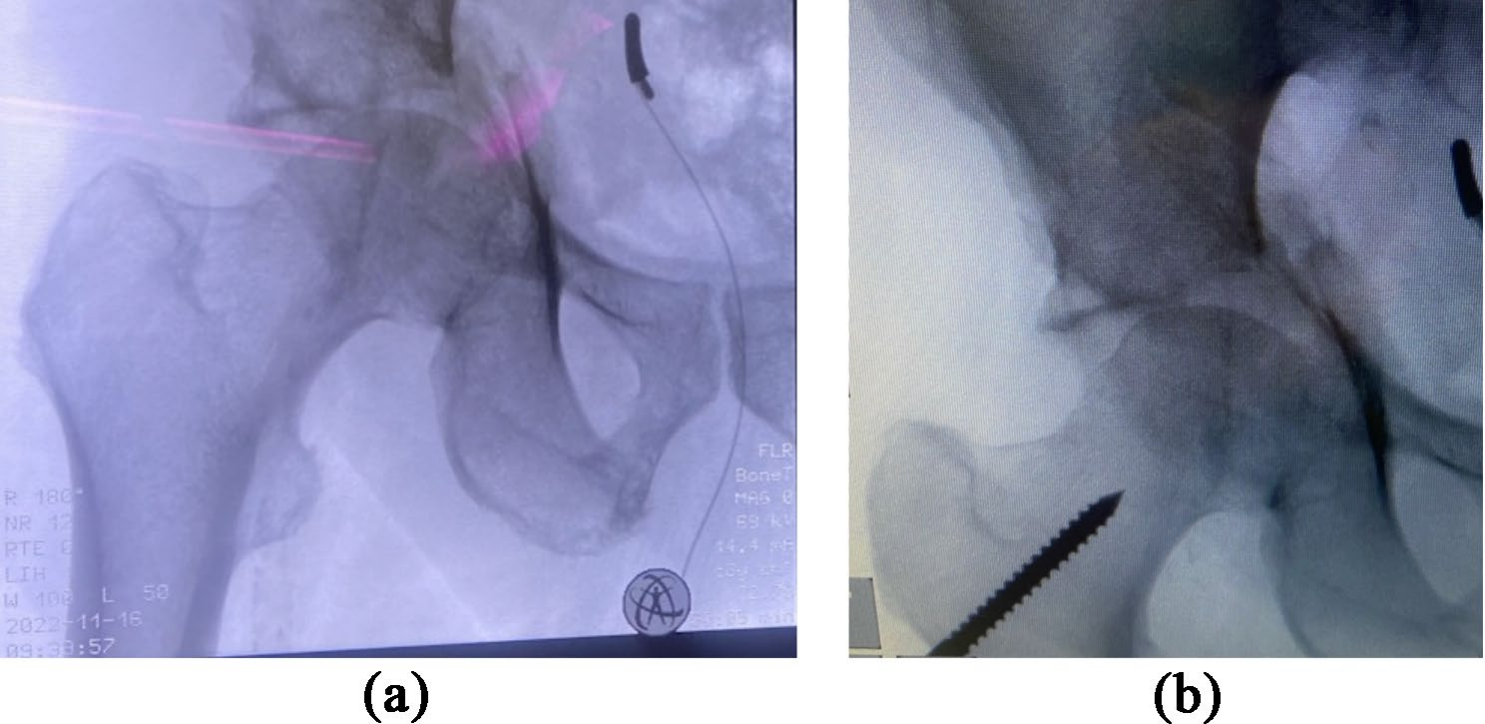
The left image (3a) displays the intraoperative X-ray image without repositioning. The right image (3b) shows the initial repositioning under traction applied by the Steinmann screw
